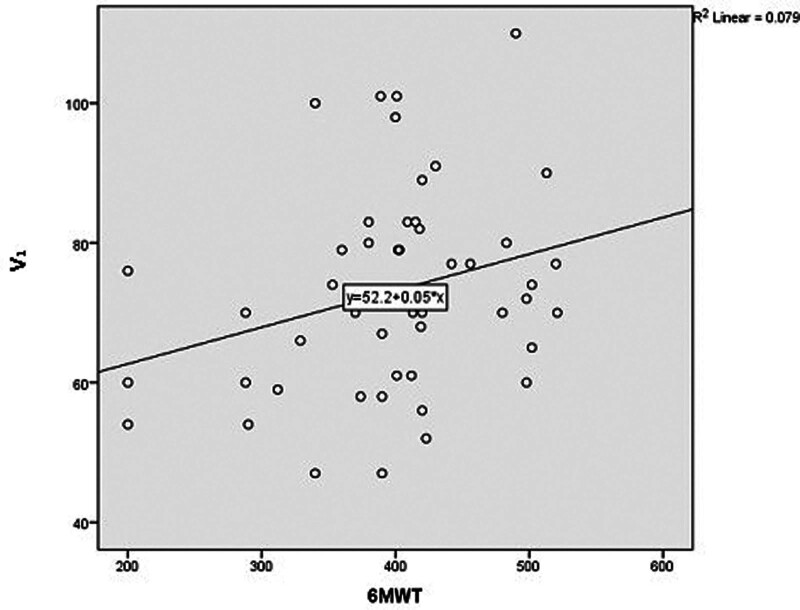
The correlation between V_1_ and 6MWT. 6MWT = 6-min walk test, V_1_ =  first pulmonary valve pulse Doppler velocity.
